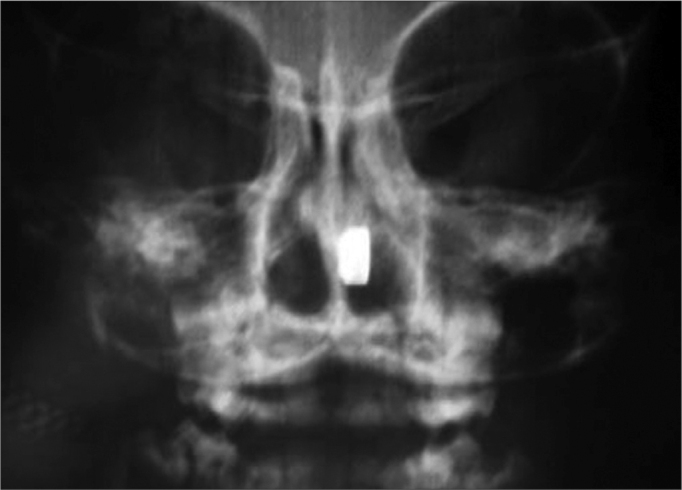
Simple fronto-nasal and side view x-rays.
